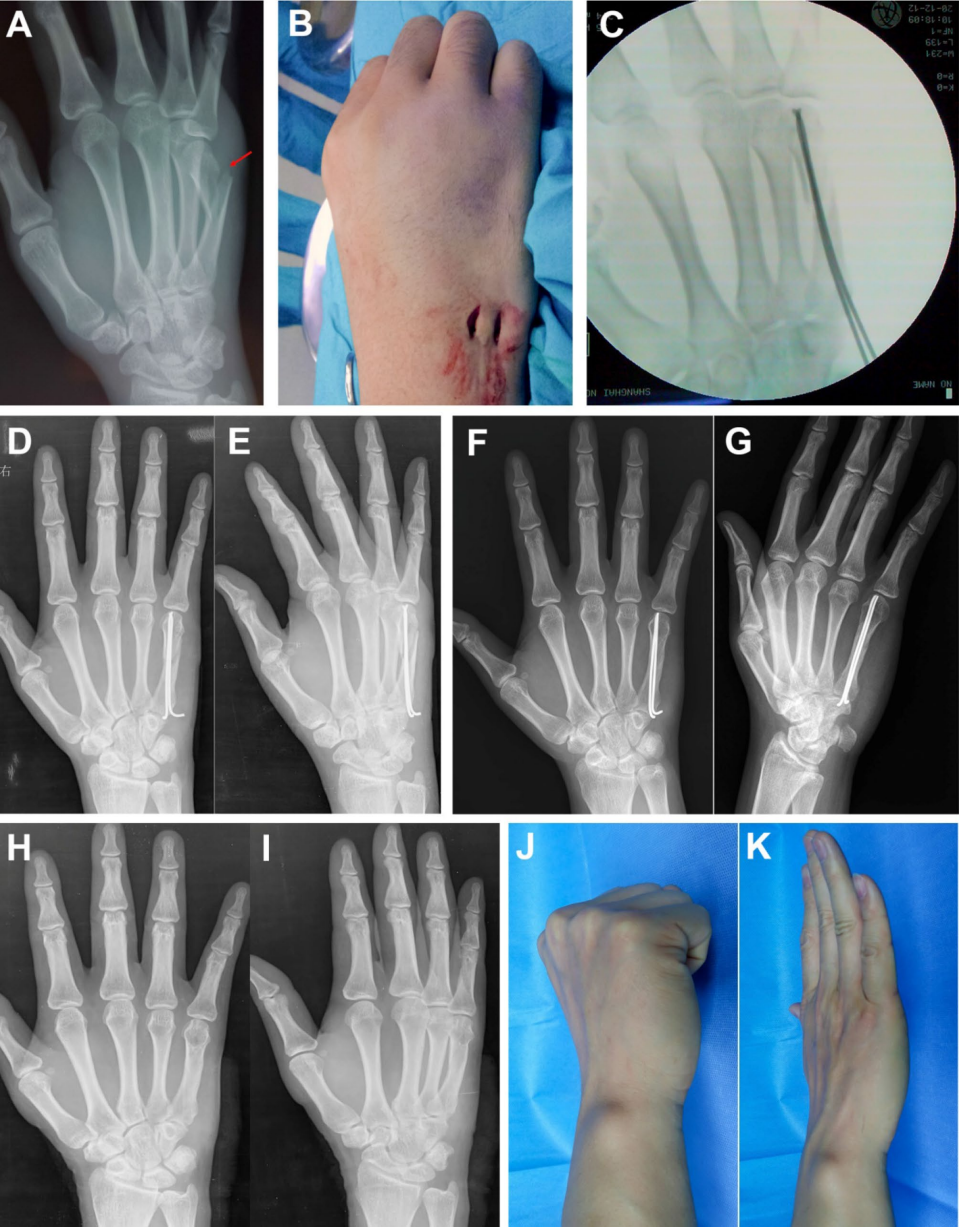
Clinical case of an FMNF treated by dual AEIMNs. Preoperative oblique view radiograph (A). Intraoperative photograph showing two incisions in the metacarpal base (B). Intraoperative fluoroscopy (C). Postoperative radiographs showing good reduction of the fracture on the anteroposterior view (D) and oblique view (E). Radiograph at 8 weeks after the operation shows union of the fracture (F, G). Radiograph at 6 months after removal of the nails (H, I). Photographs of the hand in flexion (J) and extension (K) at 12 months after operation.
